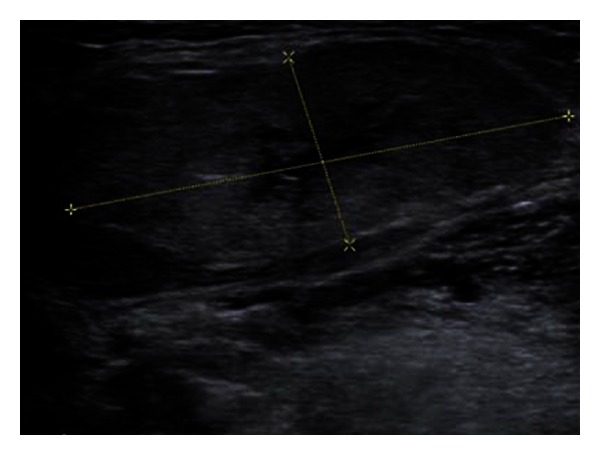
Gray scale ultrasonography of the submandibular gland (right side). The gland is enlarged (total volume around 12 cmc) with well-defined delineation, inhomogeneous structure, hypoechoic area in the center (belongs to the hilum of the gland), and hyperechoic areas under the capsule (belong to the parenchyma).
